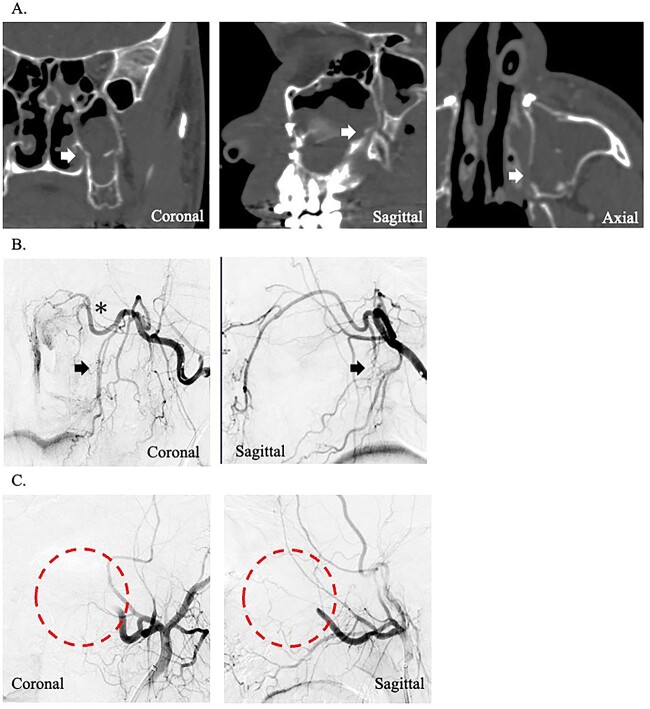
(A) Postoperative coronal, sagittal, and axial computed tomography images, which show the separated bone parts of the left maxilla (arrow). (B) Coronal and sagittal selective angiographic images from the left internal maxillary artery. The arrows indicate the suspected region of injury to the left descending palatine artery. The asterisk indicated the left sphenopalatine artery. (C) After embolization and coronal and sagittal selective angiographic images. The circle indicated the disappearance of the vascular flow area.
